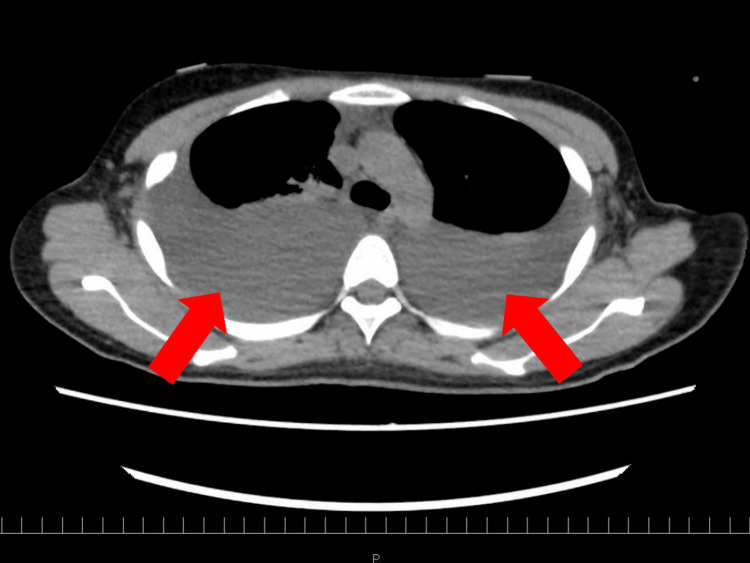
Non-contrast chest CT image at the time of admission showing large bilateral pleural effusions
